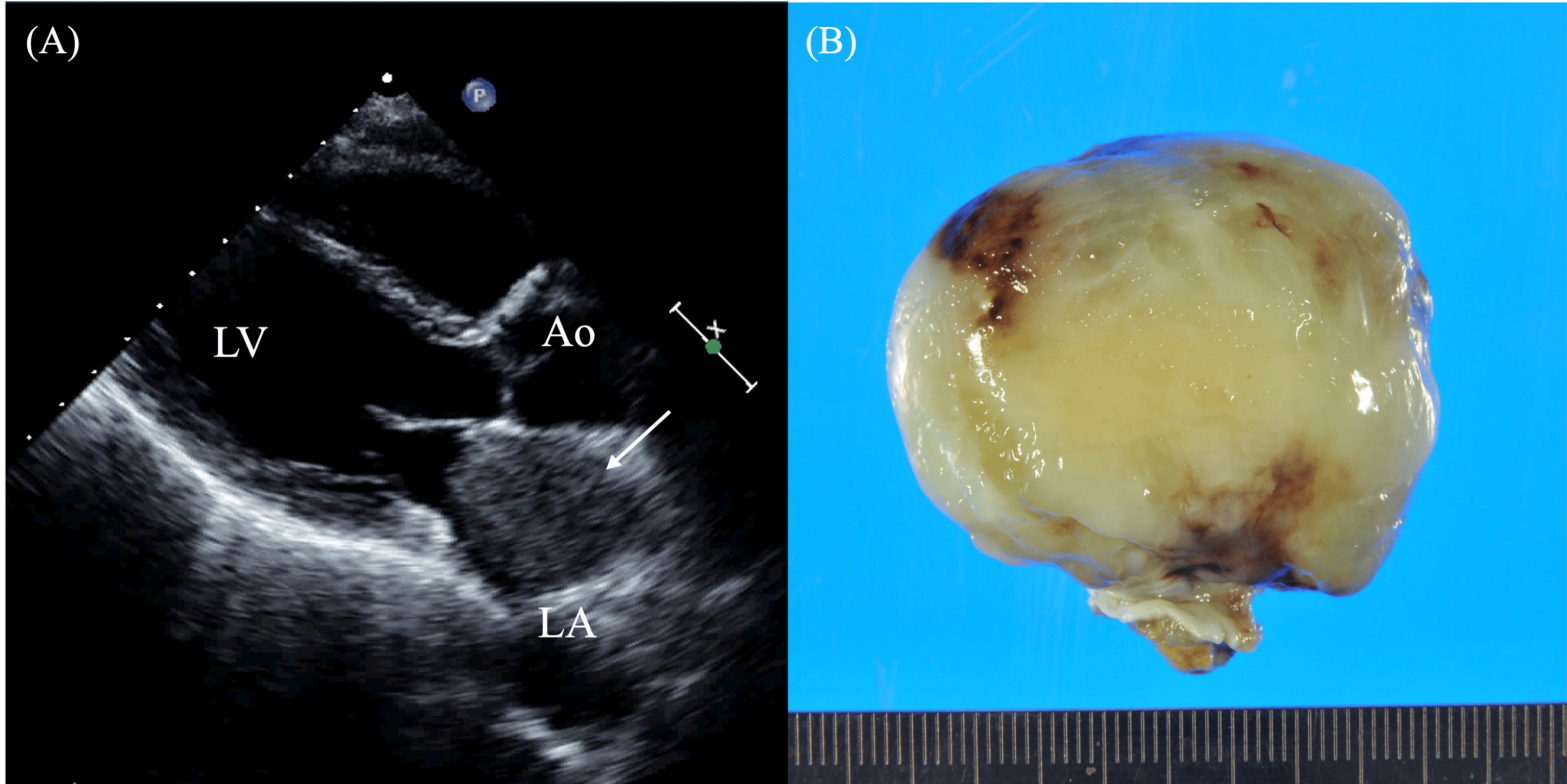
(A) UCG showing a large, mildly heterogeneous mass pedunculated from the atrial septum of the left atrium (arrow) on admission. (B) The excised tumor appeared soft and mucinous with a smooth surface (Case 1). UCG: ultrasound cardiography, LA: left atrium, LV: left ventricle, Ao: aorta.
